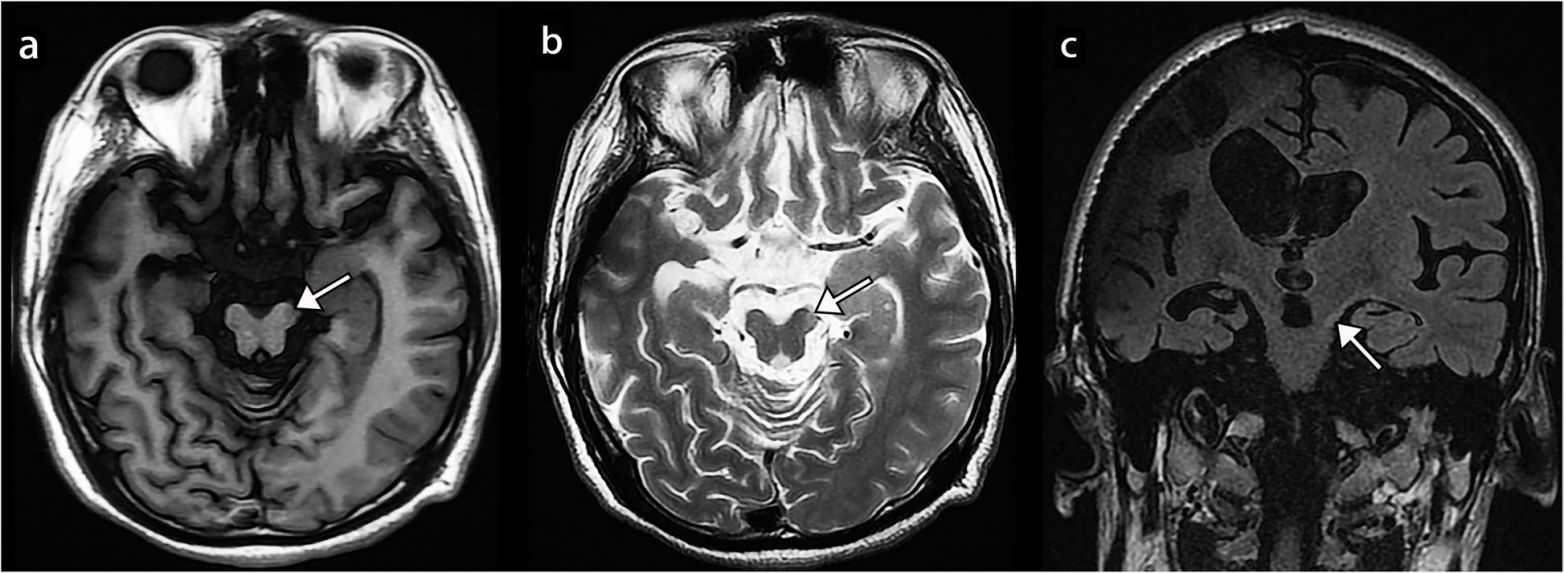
Magnetic resonance imaging displayed a subtle lesion (white arrow) in the left cerebral peduncle probably associated with the Kernohan-Woltman notch phenomenon. a Axial T1-weighted sequence. b Axial T2-weighted sequence. c Coronal FLAIR sequence also showed a right frontal lobe encephalomalacia and a moderate communicating hydrocephalus
